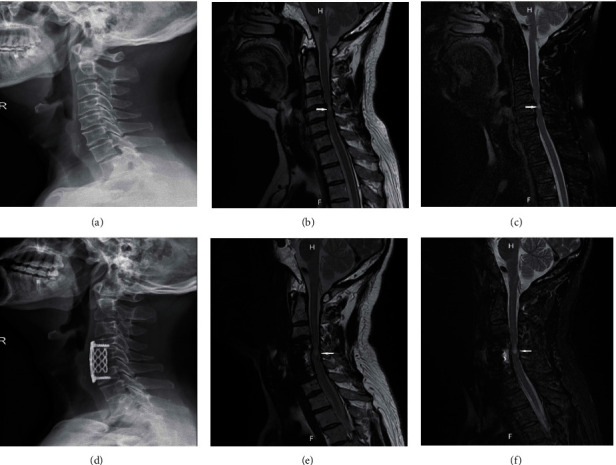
An MRI T2WIHS patient (M, 62 y) with CSWSCC presented with postoperative spinal cord IRI. (a–c) Preoperative cervical X-ray, MRI T2WI, and MRI T2 lipid suppression images, respectively, with MRI T2WIHS indicated by the white arrows. (d–f) Postoperative relative images, respectively.
